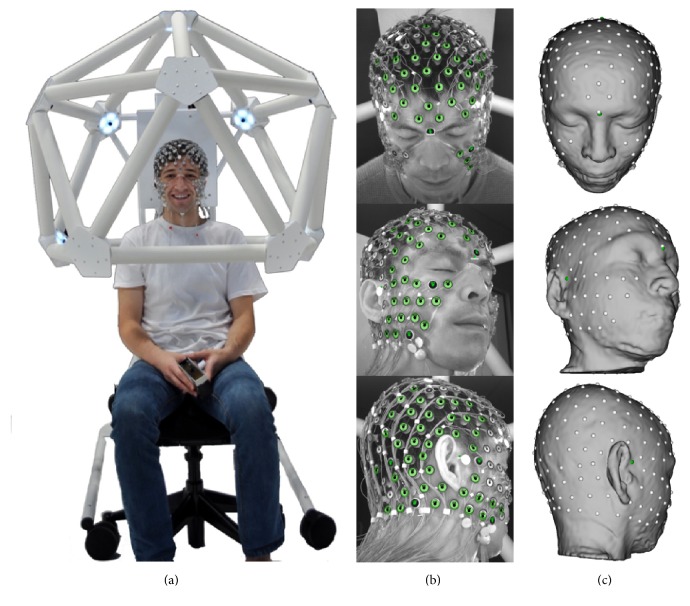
(a) Geodesic Photogrammetry System (GPS). (b) Identification of EEG sensors in the GPS software. (c) Registration of EEG sensors with the MRI head surface in BrainK.
